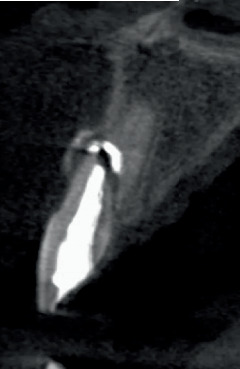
The sagittal CBCT section at 17 years of Tooth 2.1 shows displacement of the coronal fragment and the presence of a radiolucent area between the two tooth fragments.
